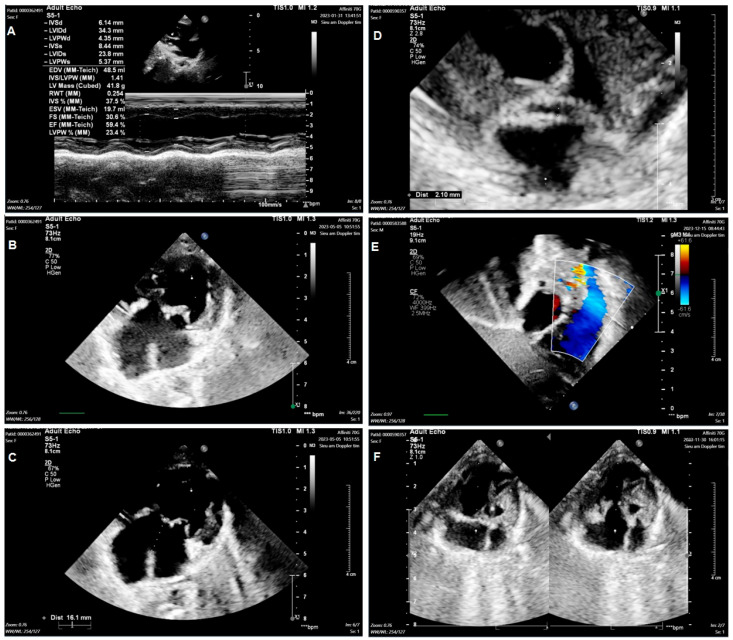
Echocardiogram in the patient with double outlet right ventricle and in the patient with hypoplastic left heart. Including the image of assessment of Ventricular function (A); Double outlet right ventricle (B); Atrioventricular septal defects (C); Hypoplastic left heart (D); Pulmonary stenosis (E); Aortic stenosis (F).
